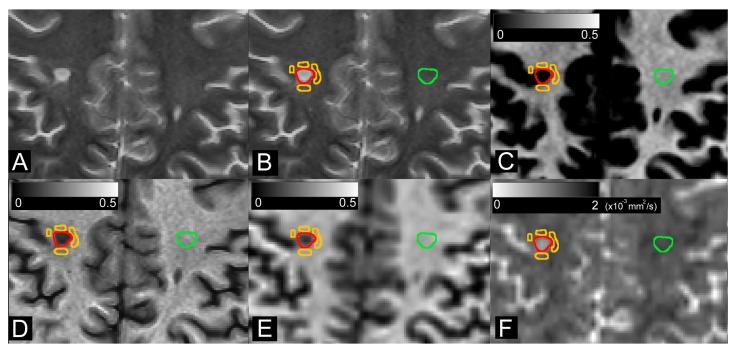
Synthetic T2-weighted images (A) were used for semi-automated segmentation of plaque (red), periplaque (orange), and contralateral normal-appearing white matter (green) (B). Regions-of-interest were copied and pasted onto SyMRI_MVF_ (C), T1w/T2w (D), MTsat (E), and RD (F) maps.
